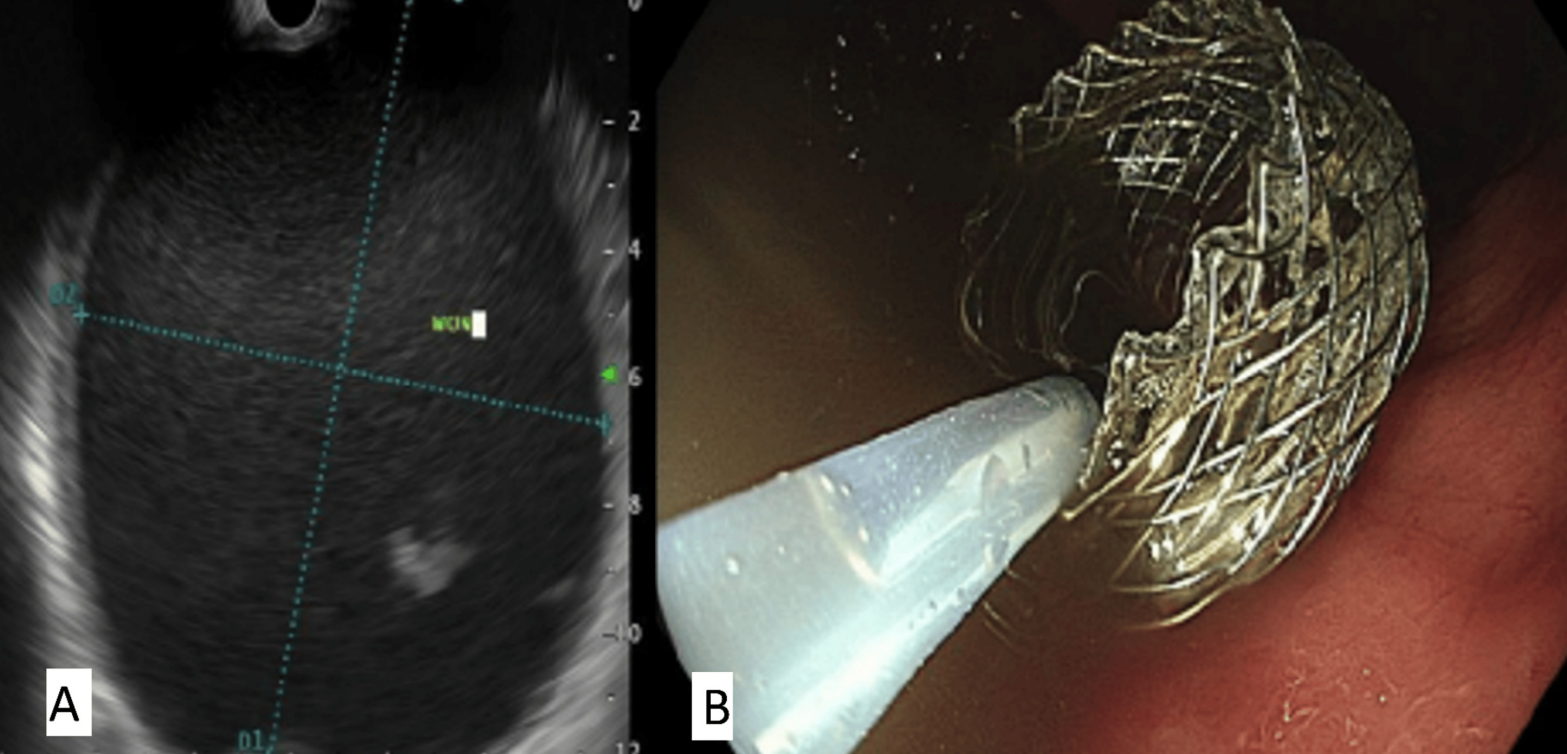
(A) EUS view of the WON on the right. (B) Endoscopic view of LAMS on the left. EUS: endoscopic ultrasound; WON: walled-off necrosis; LAMS: lumen-apposing metal stent.
